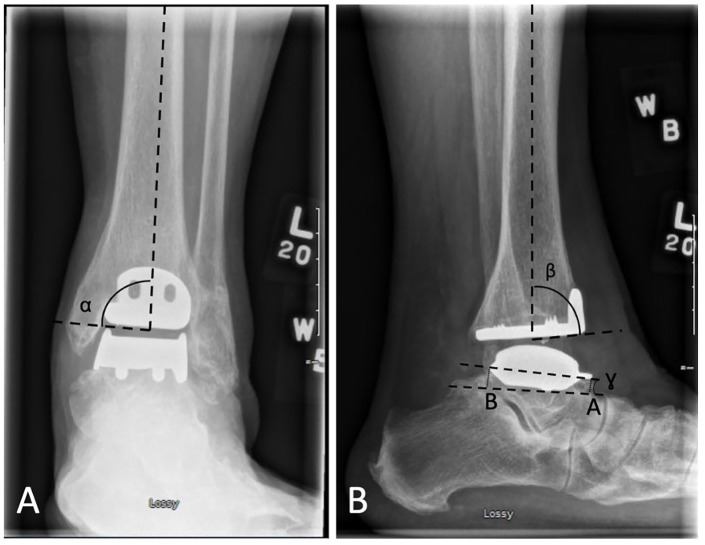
Radiographic alignment measurements (A) alpha angle, (B) beta angle, gamma angle, and A and B distances.
